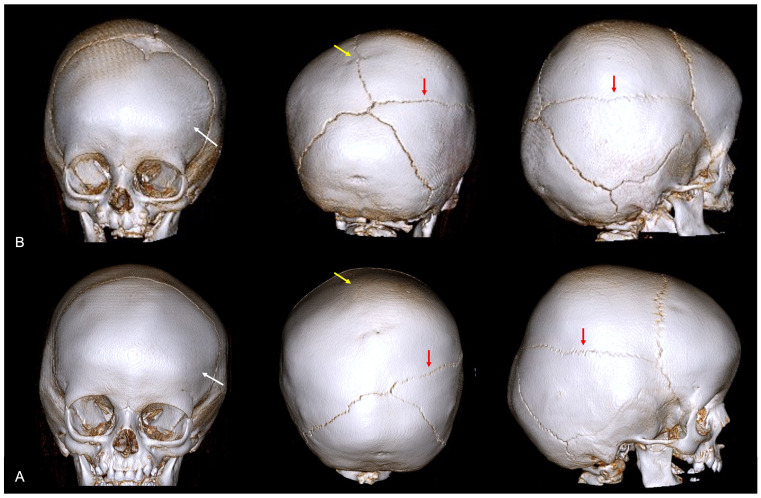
A 3D CT image of complex nonsyndromic CRS in a 6-month-old patient diagnosed with skull deformity. (A) Right frontal eminence asymmetry (white arrow) and minor left posterior plagiocephaly, with incipient sagittal synostosis (yellow arrow) and the presence of a right parietal accessory suture (red arrow). Subsequent 1-year follow-up examination (B) confirmed mild skull deformity due to complete fusion of the sagittal suture, not resulting in classic scaphocephaly due to patency of the PA suture.
